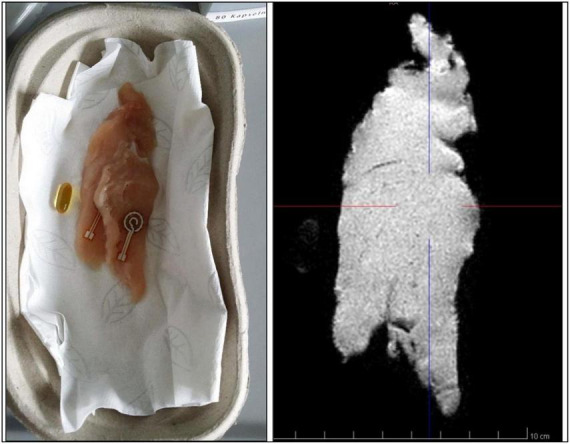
Graphene nanoplatelets do not generate artifacts during magnetic resonance (MR) Imaging. The left panel shows a photograph of an arrangement with two graphene nanoplatelet electrodes placed on top of, or between, two layers of chicken meat, alongside a vitamin capsule as a reference, all arranged in an MR-compatible paper tray. Note that in the MR image shown in the right panel, only the chicken meat is visible; the electrodes are not detectable, indicating the absence of imaging artifacts. The electrodes consisted of circular leads with diameters of 18 mm and 7 mm, a thickness of 1.5 mm, and lead wires measuring 20 mm in length.
